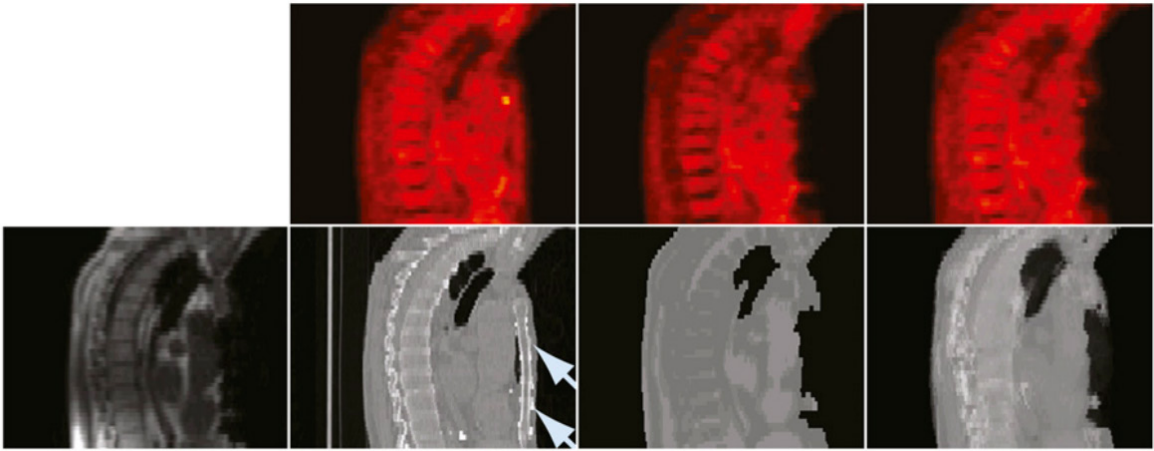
Patient with metal implant in sternum. Bottom row, from left to right: in-phase MR image; CT image, with arrows indicating location of metal implant; pseudo-CT from 5-class MR image segmentation; and AT&PR-based pseudo-CT. Top row: corresponding PET images reconstructed using pseudo-CT images for AC. This research was originally published in JNM. Hofmann et al. “MRI-Based Attenuation Correction for Whole-Body PET/MRI: Quantitative Evaluation of Segmentation- and Atlas-Based Methods ”. *J Nucl Med*. 2011;52:1392–1399. ^©^ SNMMI.^61^
